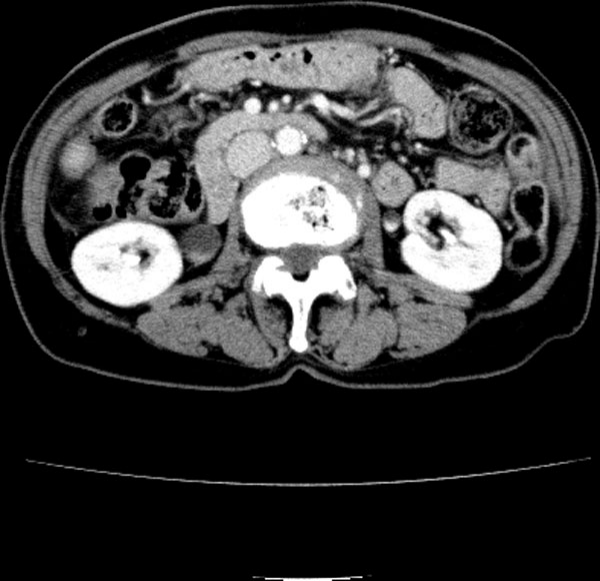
Axial CT scan obtained with IV contrast material in 76-year-old female benign subject.
